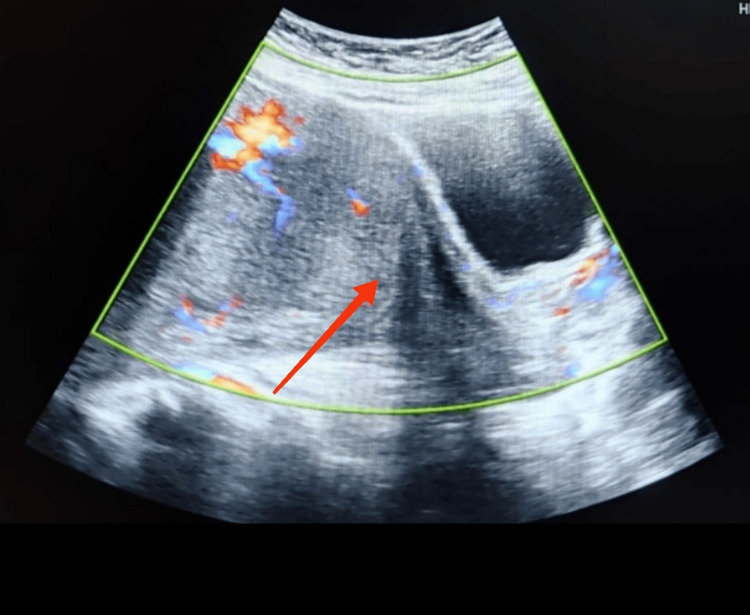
Ultrasonography of central placenta previa The arrow shows the placenta completely covering the cervical os.
